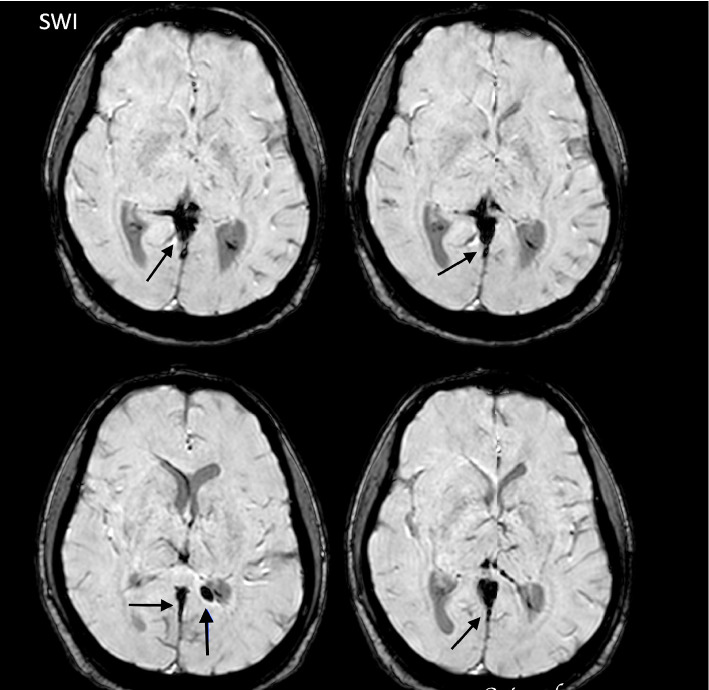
MRI brain showing thrombosis of the straight sinus.
